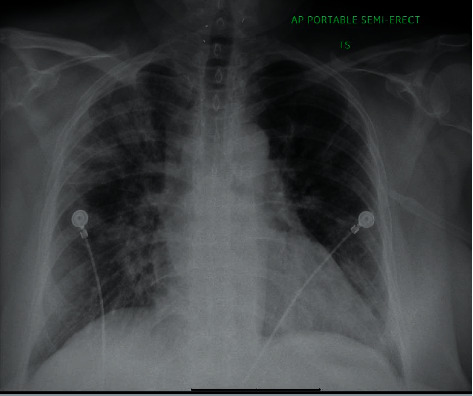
Chest X-ray showing an improvement in bilateral infiltrates.
